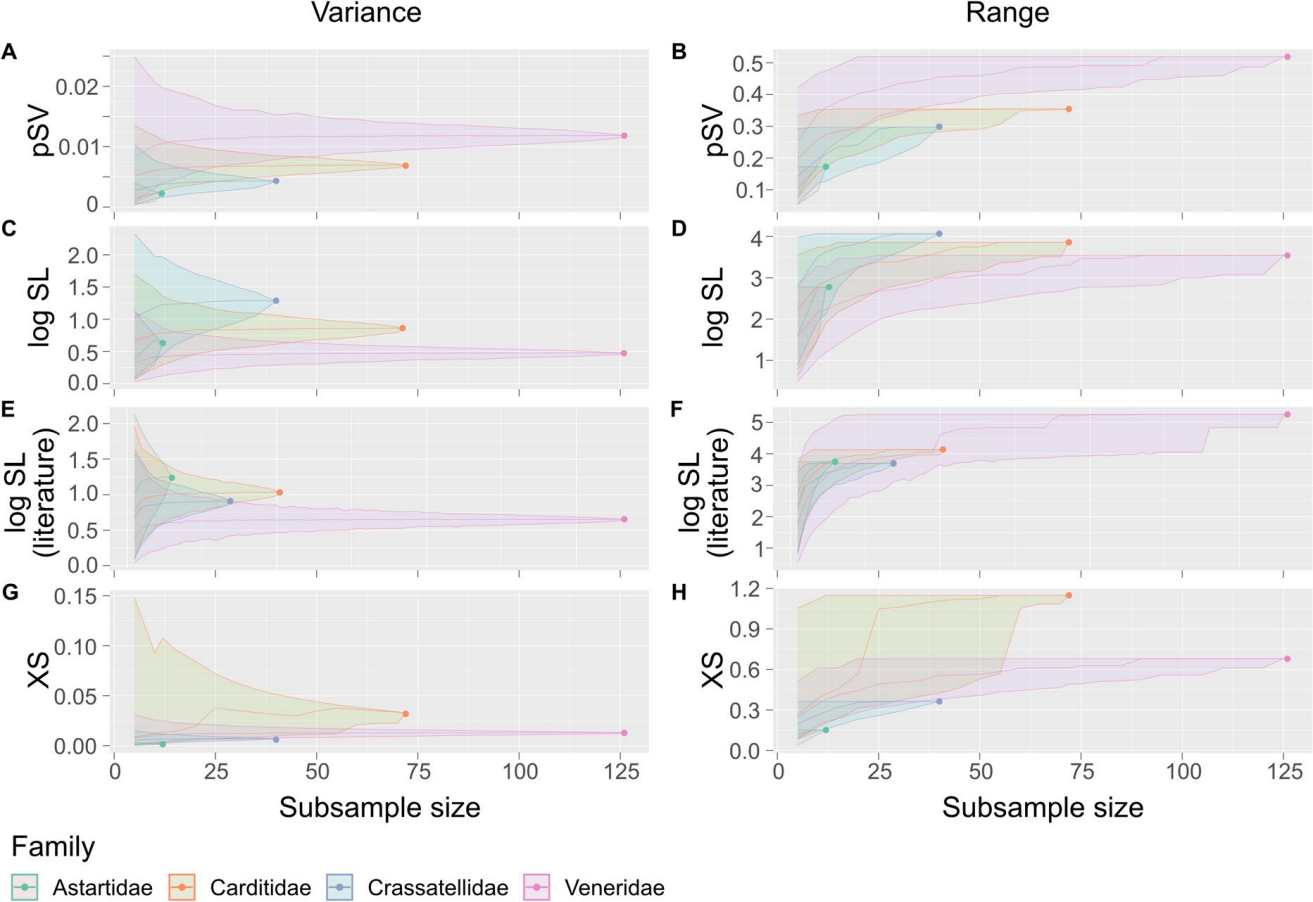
Rarefaction curves for the median disparity (two different metrics: variance and range) and 95% confidence intervals (shaded area) of pSV of the species analyzed here (A, B); logSL of the species analyzed here using CT, (C, D); logSL calculated from a literature compilation by Huang et al. (2023) of body lengths for all species (E, F); and XS (G, H) of the species analyzed here. Measured disparity for the full sample of each family is shown by a point at the far right of the confidence envelope
